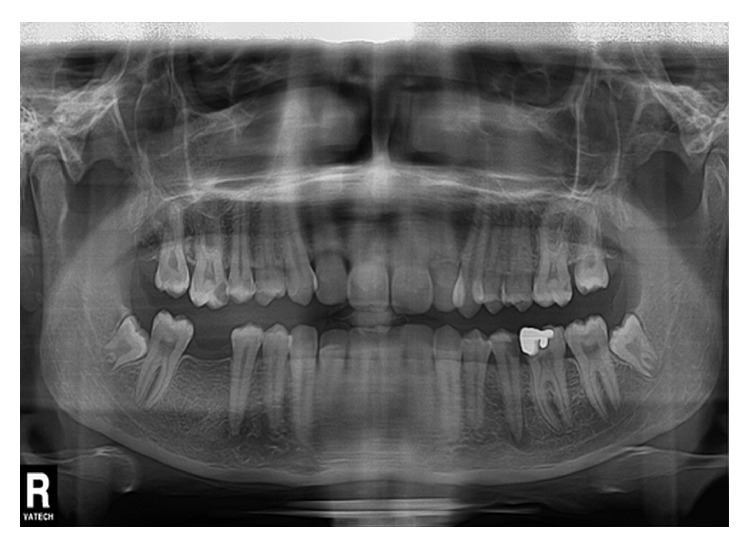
Preoperative panoramic radiograph of the case. Root of mandibular left second premolar seems to be in close proximity to mental nerve.
